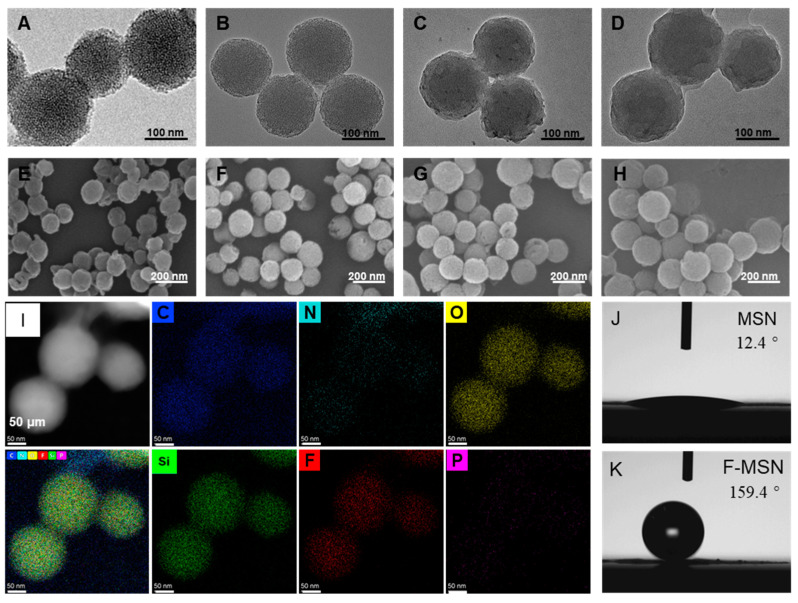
Characterization of NPs. (A–D) SEM and (E–H) TEM of the MSN, F-MSN, F-MSN-DOX and F-MSN-DOX@RBC; (I) SEM image, energy dispersive X-ray (EDX) elemental mapping of C, N, O, SI, F, and P of F-MSN-DOX@RBC; (J,K) Contact angle of films made from MSN and F-MSN.
